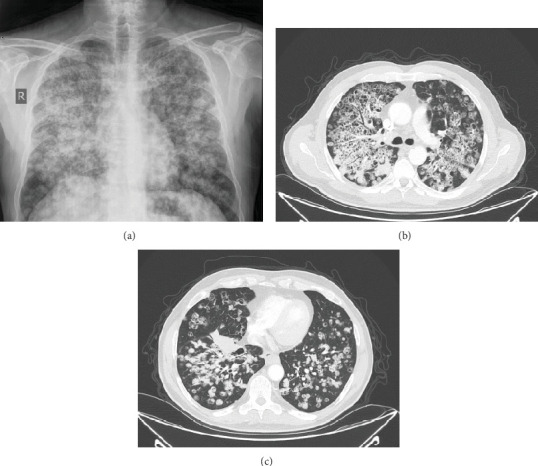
(a) Chest x-ray: diffuse bilateral reticulonodular opacities with upper and midlung consolidations, indicating extensive parenchymal involvement. (b, c) Axial chest CT: multiple cavitary nodules with irregular margins and architectural distortion.
